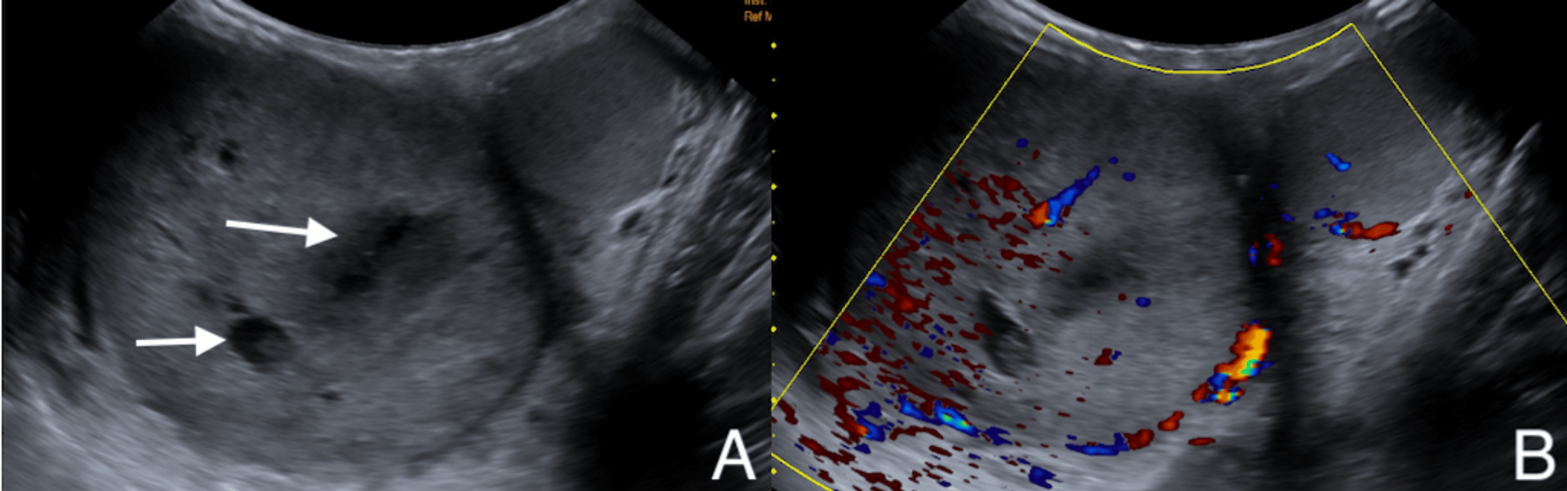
Scrotal ultrasound of an enlarged, heterogeneous right testicle with mixed echogenicity (A) and spectral doppler flow demonstrating increased vascularity (B)
